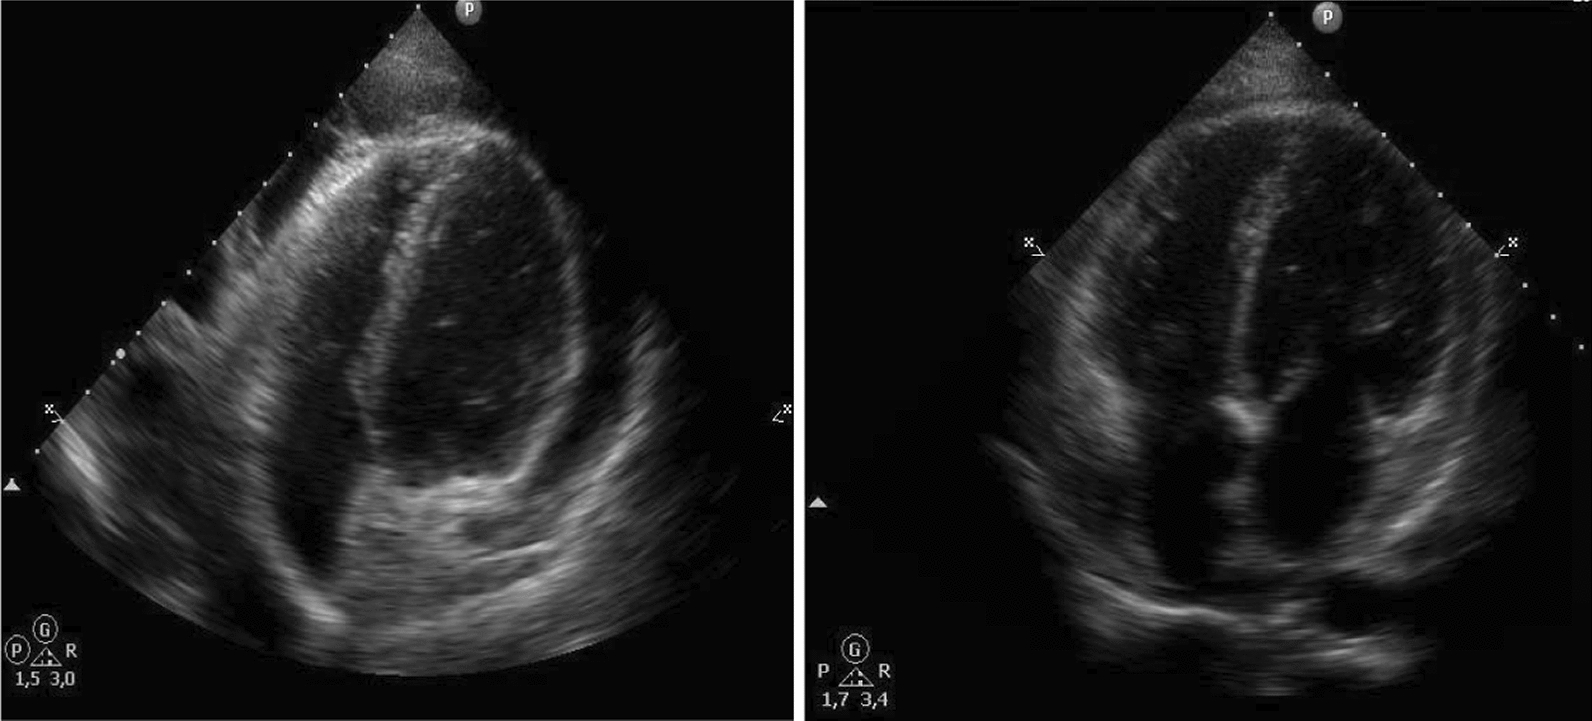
Echocardiography in a patient with PL and associated chylous pericardial effusion before and after 8 months of treatment with sirolimus
